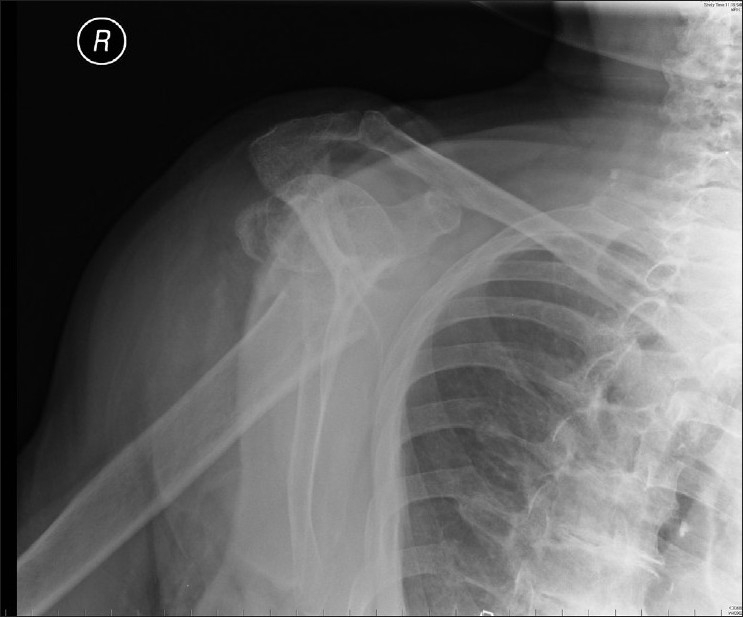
Plain lateral scapula radiograph of right shoulder. A ‘Neer’ 2-part fracture is seen
